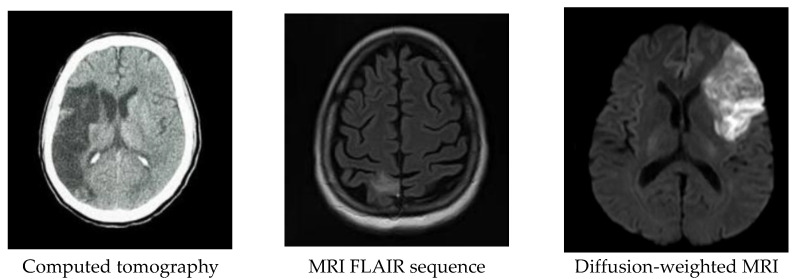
Neuroimaging in acute ischemic stroke of different patients. On computed tomography, infarct is seen as a hypointense region (left). With MRI, signal contrasts between different tissues can be amplified using different pulse sequences. With fluid attenuated inversion recovery (FLAIR), the infarct is depicted as a bright signal against surrounding brain tissue as well as the dark signal-suppressed cerebrospinal fluid (center). With DWI, the infarct tissue exhibits less signal decay as water diffusion becomes restricted, which shows up as a hyperintense area (right).
